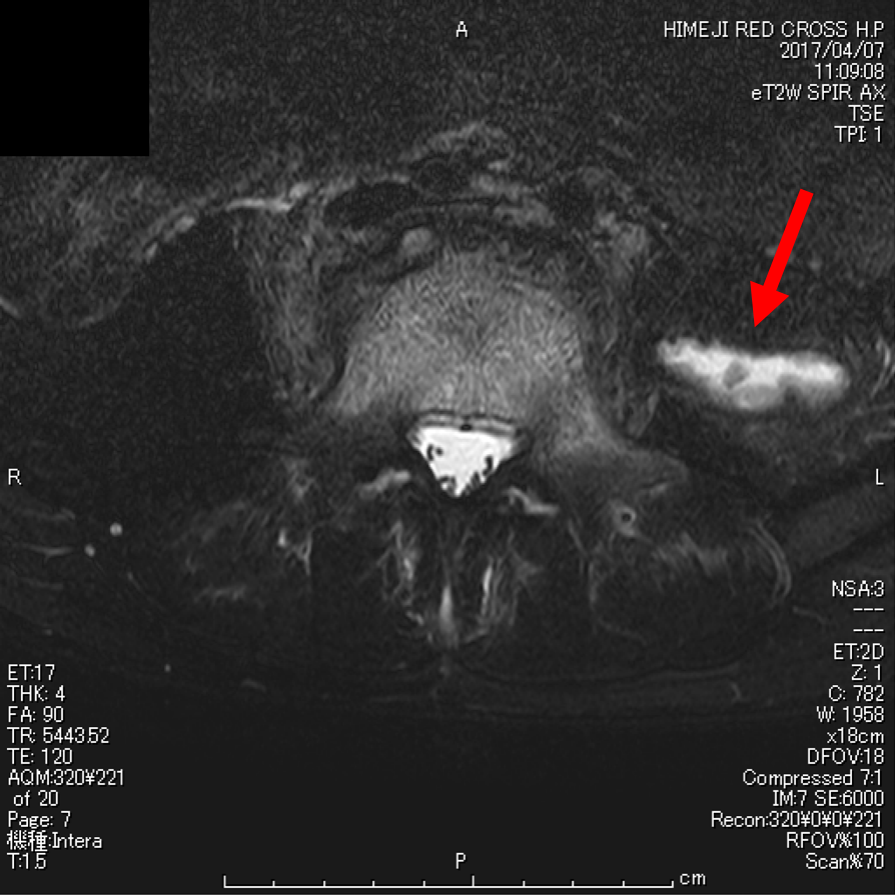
MRI after 2 weeks showed newly diagnosed left psoas abscess
